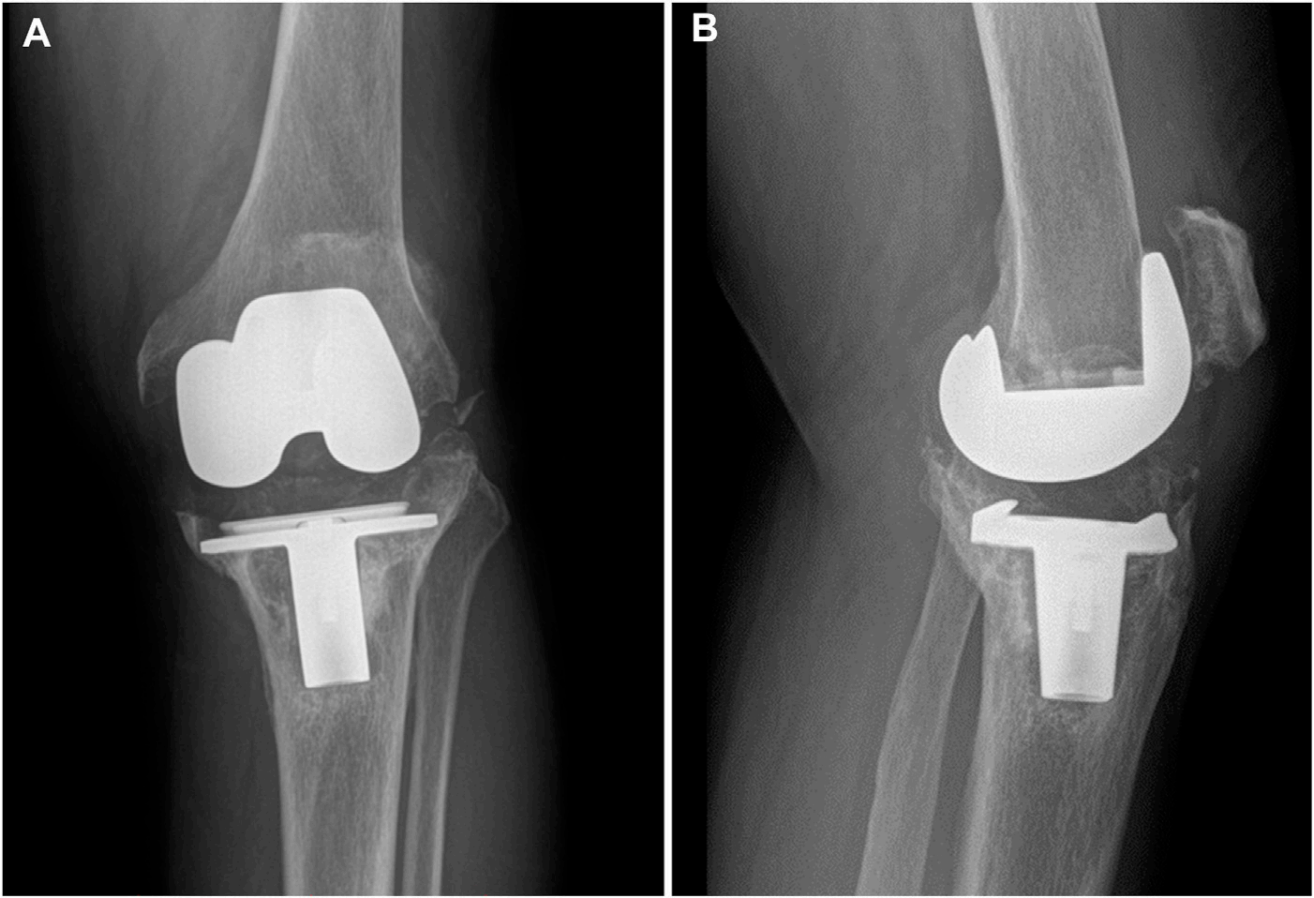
Preoperative radiographs of the typical case showing collapse of the tibial plateau. (A) Coronal view; (B) sagittal view.
